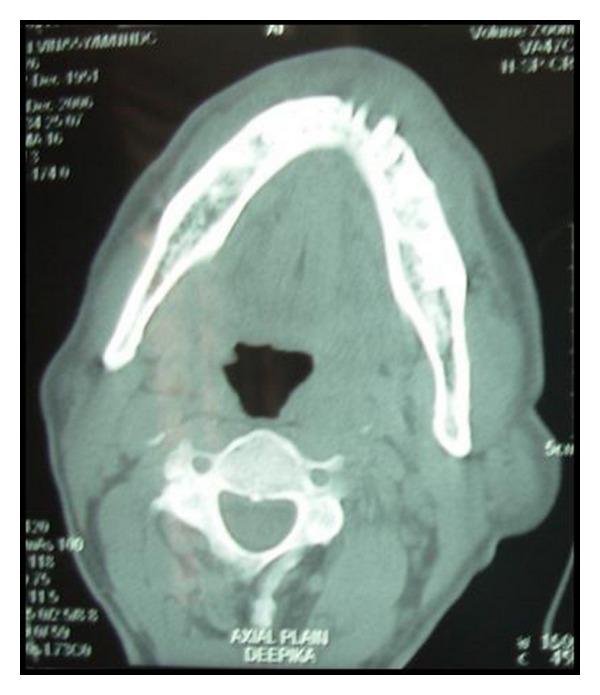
Erosion of the anterior cortex of the left body of mandible seen on the axial CT scan bone window.
